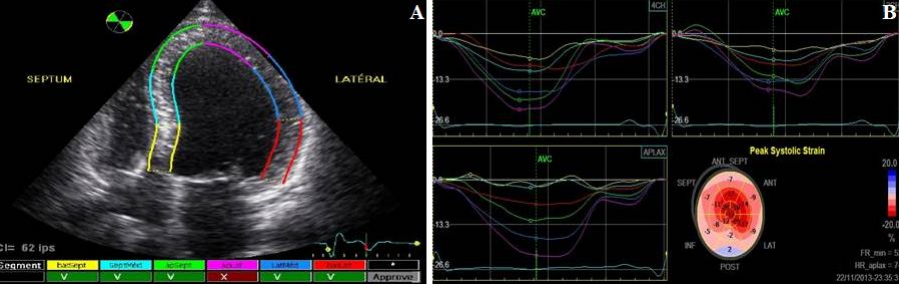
demonstration of two-dimensional speckle tracking imaging (2D-STI) analysis; after the reference points are set, the software shows the endocardial border tracking in different views; a) the apical 4-chamber view (the endocardial tracking is performed automatically through the entire cardiac cycle by 2D-STI); b) the LV longitudinal time velocity curves obtained from the apical 4- 3- and 2- chamber views in hemodialysis patients
